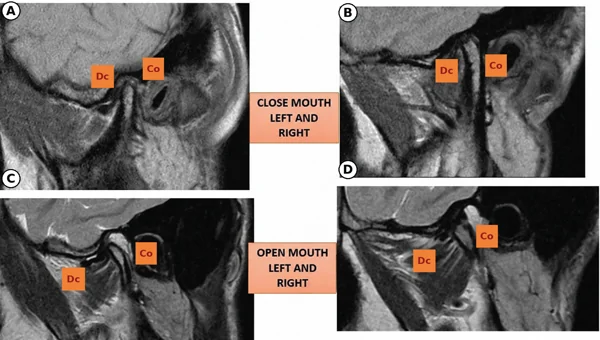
Sagittal magnetic resonance imaging (MRI) of the temporomandibular joints (TMJs) in Case 1, showing the right and left joints in closed‐mouth and open‐mouth positions. (A) Right TMJ, closed‐mouth position. (B) Left TMJ, closed‐mouth position. (C) Right TMJ, open‐mouth position. (D) Left TMJ, open‐mouth position. In each panel, Dc denotes the articular disc and Co denotes the mandibular condyle.
